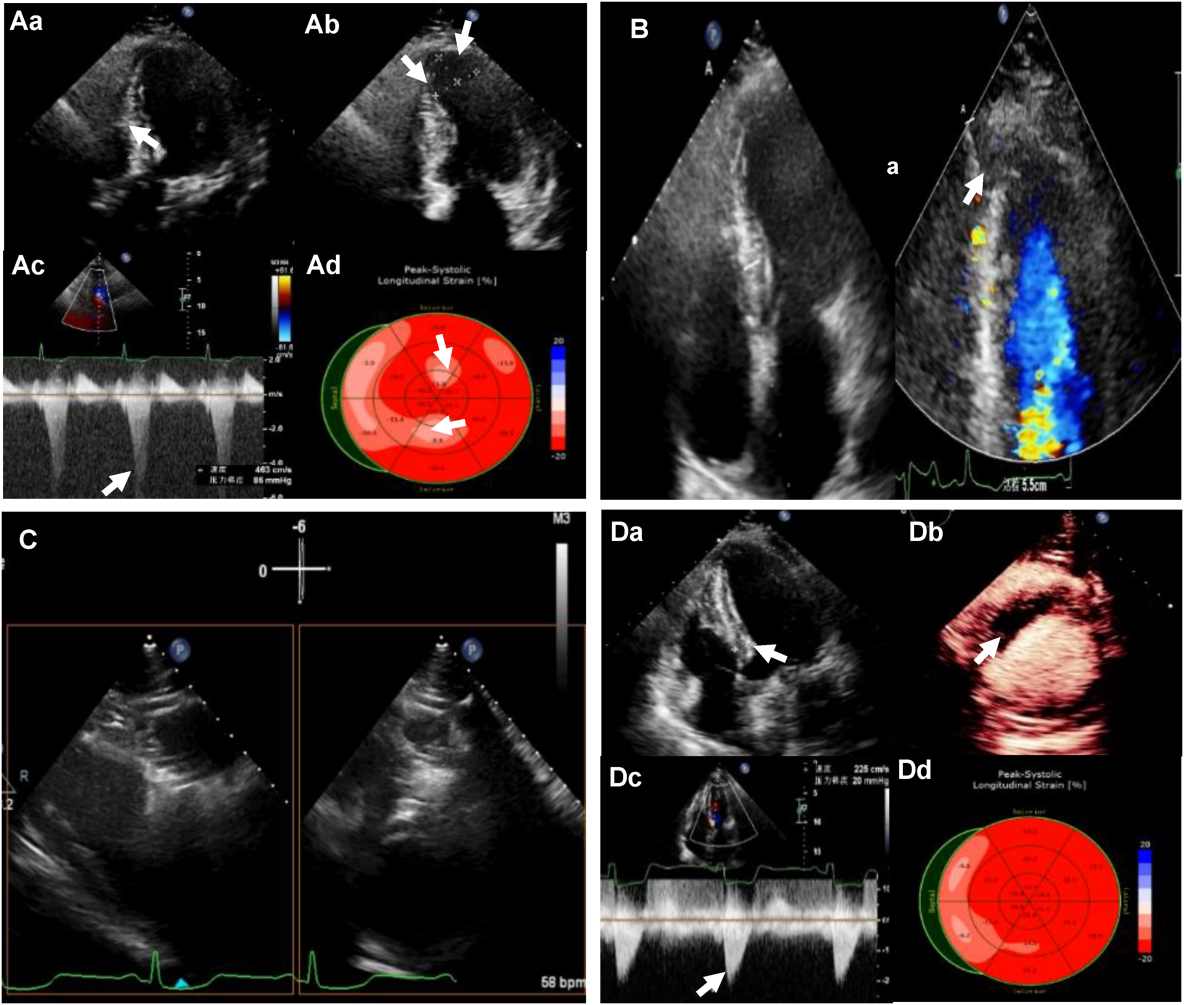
(Aa) Preoperative transthoracic echocardiography (TTE): left ventricular (LV) septal thickness of 20mm; (Ab) the extent of ventricular aneurysm; (Ac) left ventricular outflow tract (LVOT) obstruction at rest; (Ad) reduced strain value of local LV apex by strain echocardiography (SE). (B) LV apical entrance of the ablation needle guided by TTE. (C) Bi-plane showing ablation needle position. (Da) LV septal thickness of 12 mm after ablation; (Db) myocardial contrast-enhanced echocardiography (MCE) showing a contrast filling defect in the ablation zone; (Dc) LVOT gradient of 20 mm Hg after the ablation; (Dd) LV apical systolic synchrony detected by SE.
